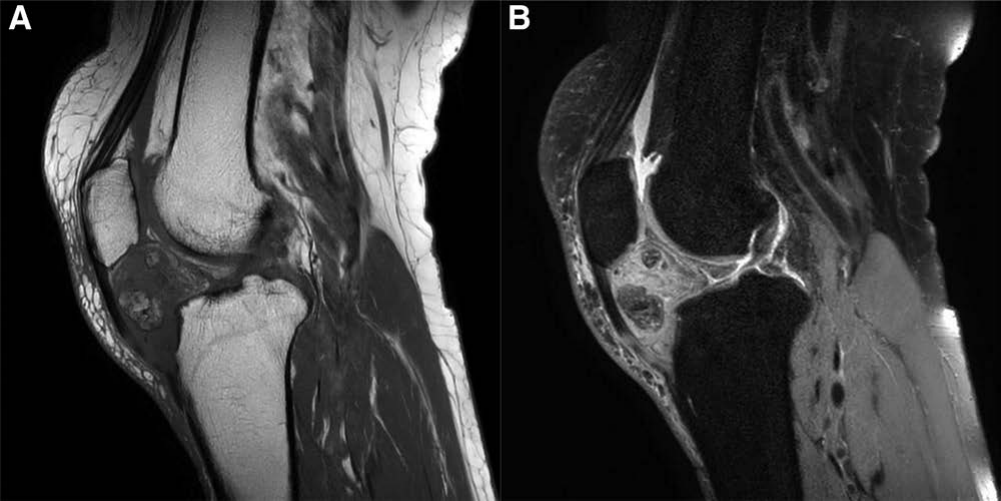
Magnetic resonance imaging (MRI) shows clearly the mass in the infrapatellar fat pad. It shows a lobulated mass. T1 image (A), T2 image (B).
